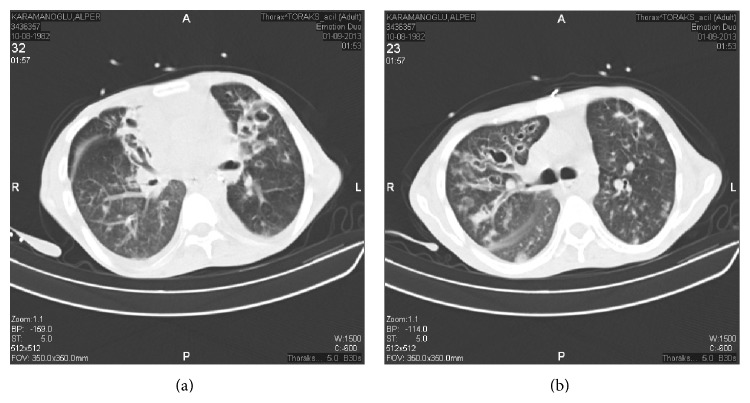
Thoracal CT of the patient shows bilateral bronchiectatic segments.
